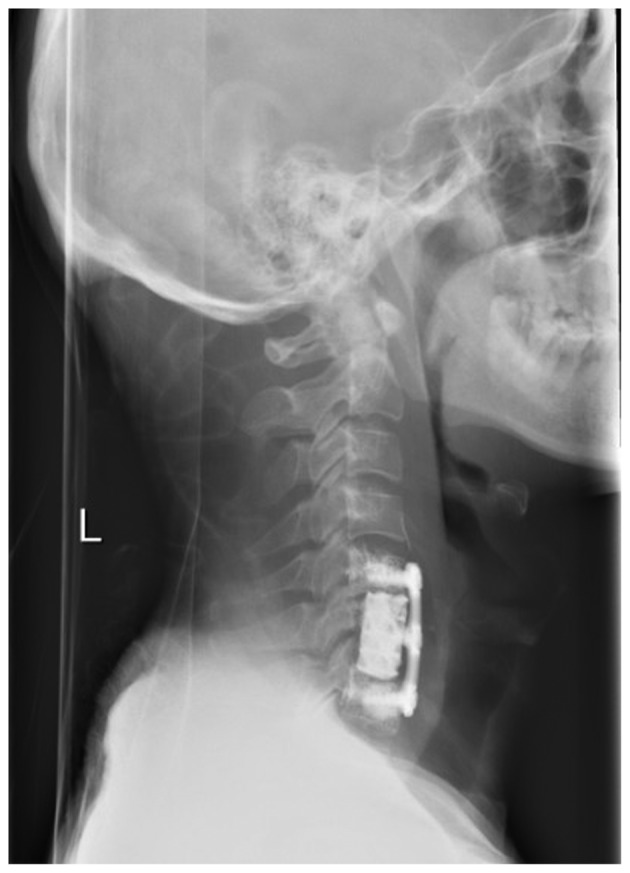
Lateral radiograph obtained 12 months after surgery revealing that no fixation failure or bone cement leakage had occurred within this time.
